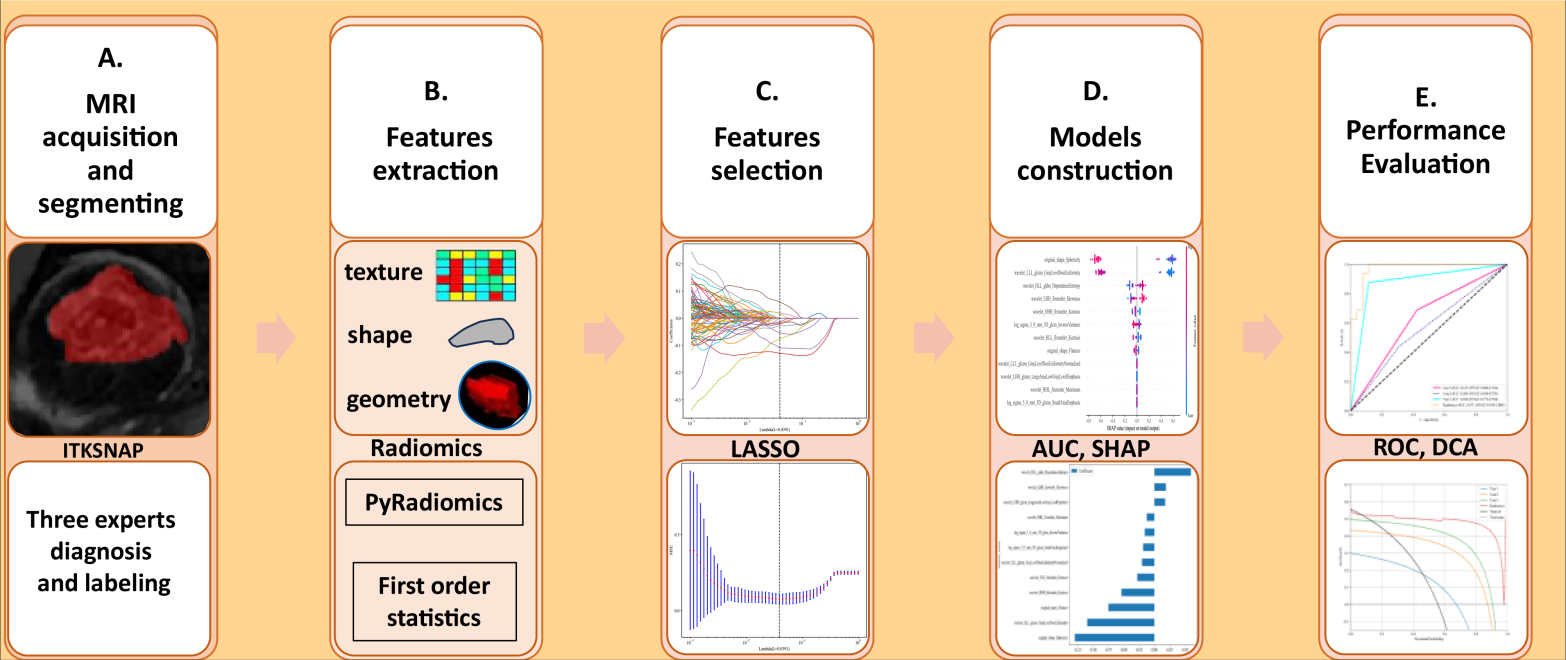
(A–E) The methodology and flow of this study, beginning with MRI data gathering and ending with performance evaluation, includes segmentation, feature extraction, selection, and the development of multiple machine learning models. AUC, Area Under the Curve; SHAP, SHapley Additive exPlanations; ROC, Receiver Operating Characteristic; DCA, Decision Curve Analysis.
